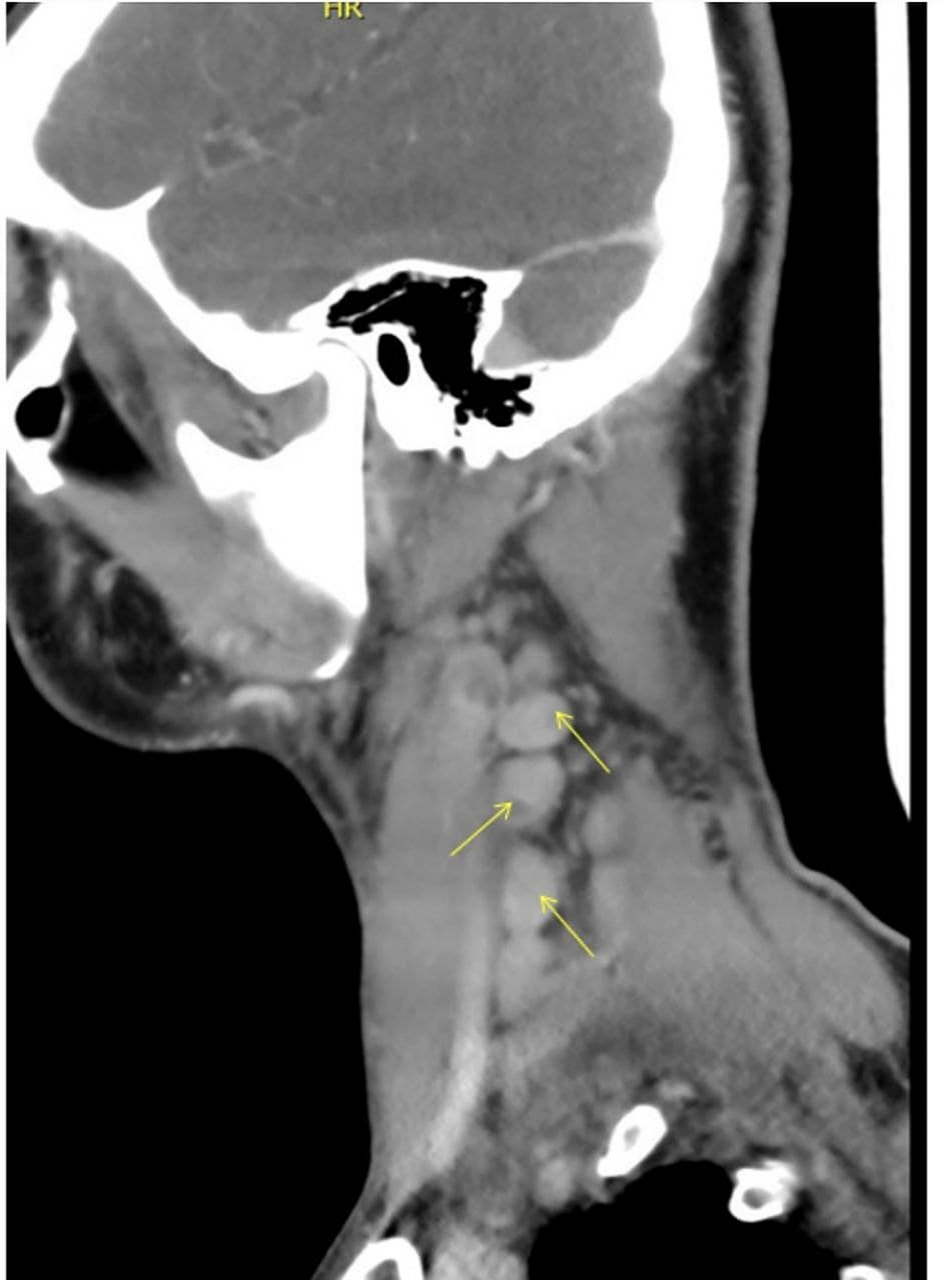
Neck CT: lateral sagittal view of right deep cervical lymphadenopathy.
